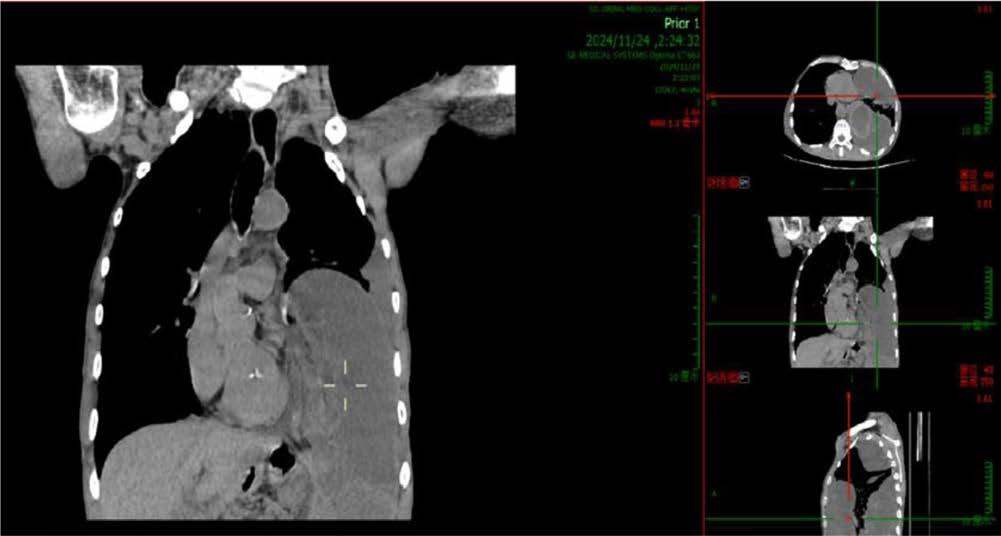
November 24, 2024, chest and abdominal CT demonstrated a left-sided diaphragmatic hernia complicated by bowel obstruction. CT = computed tomography.
